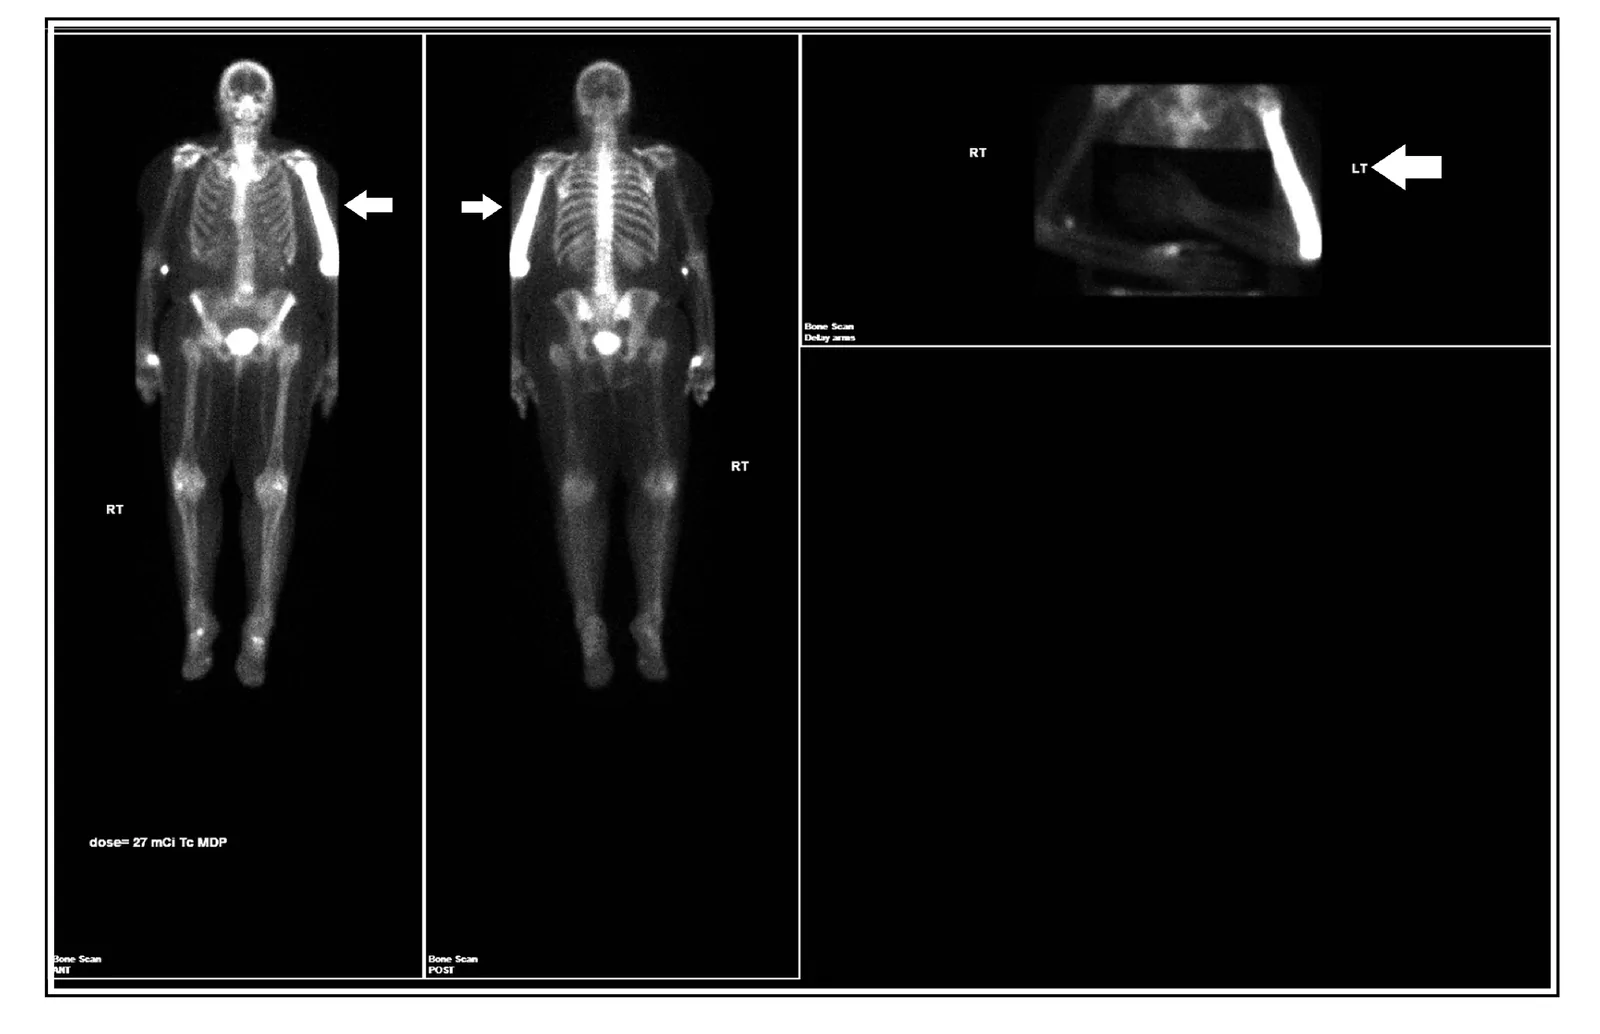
Bone scintigraphy shows marked increased activity throughout the entire left humerus (arrows), with mild increased activity in the L5 vertebral body. Created in BioRender.com by JJL.
